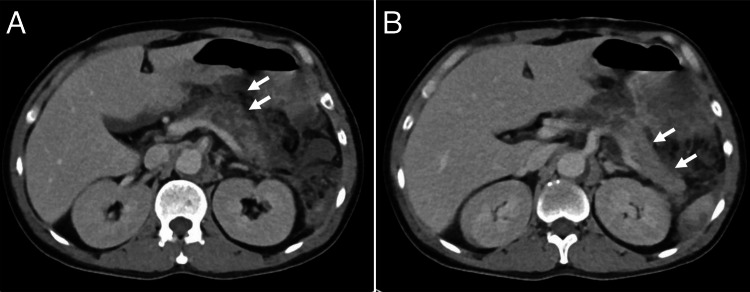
Ischemic pancreatitis of the pancreatic body caused by aortic dissection. An axial image from the contrast-enhanced CT in the equilibrium phase shows a poorly contrasted area in the pancreatic body, along with the increased density of the surrounding fatty tissue, consistent with acute pancreatitis (A). In the pancreatic tail, although the surrounding fatty tissue exhibits increased density, contrast enhancement is preserved (B).
